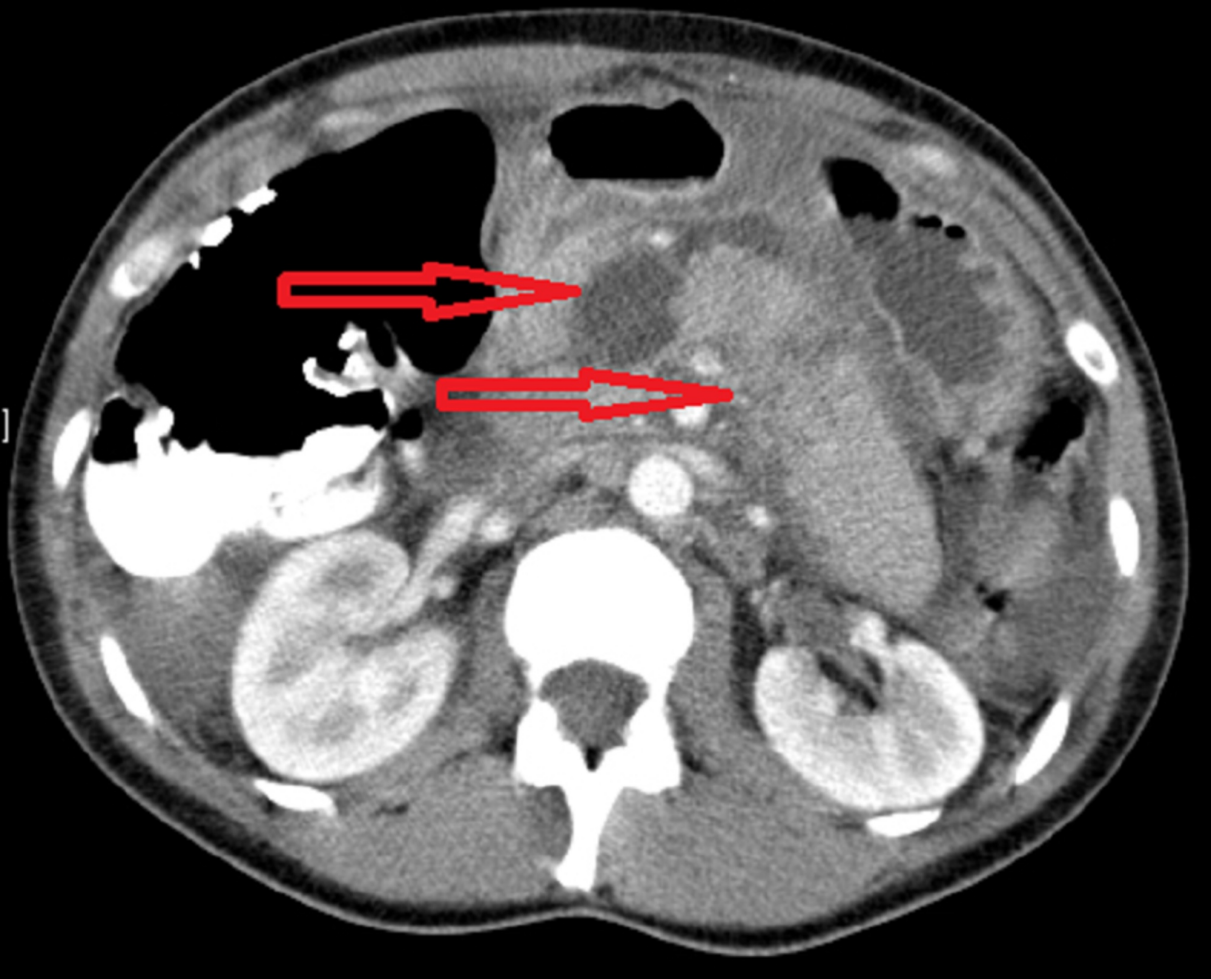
Computed tomography (CT) scan showing significant injury to pancreatic neck and body. Top arrow: neck; bottom arrow: body.
